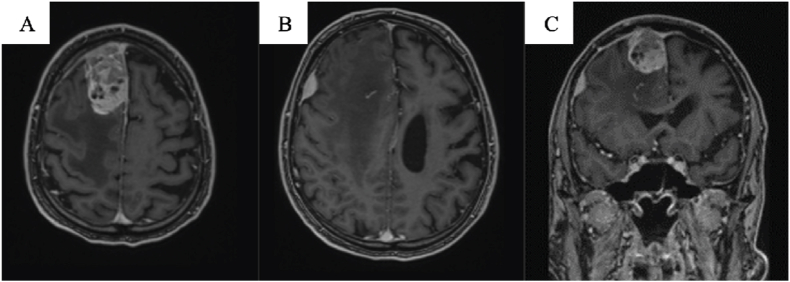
Contrast-enhanced T1-weighted images before surgery. A & B: MRI in axial plane. C: MRI in coronal plane.
